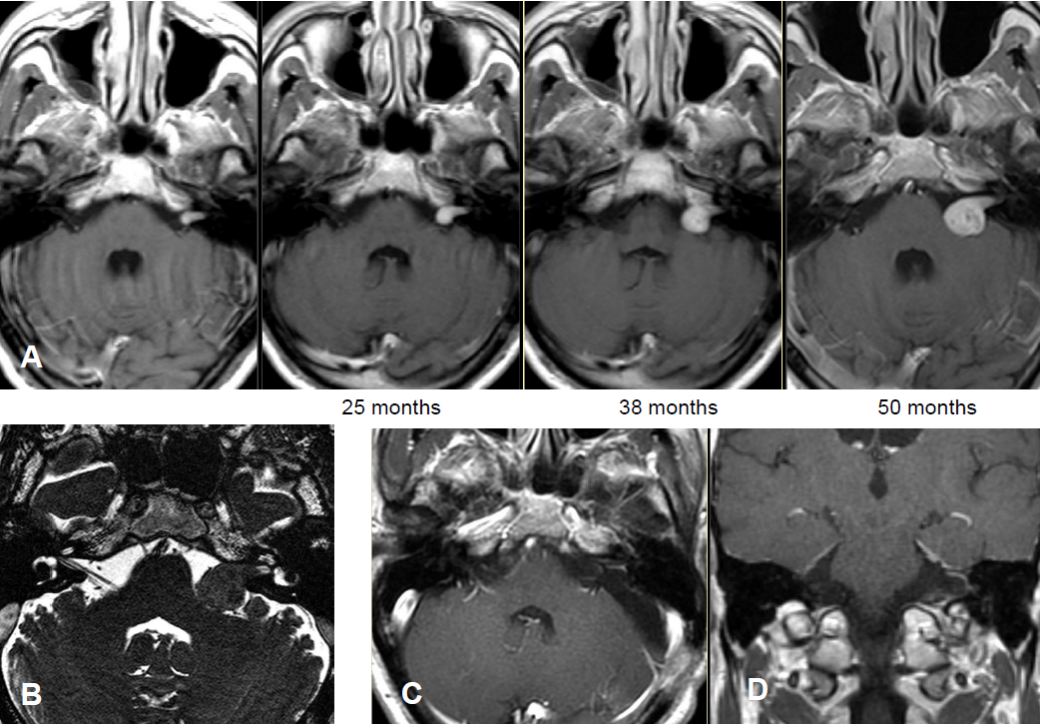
Upper row: MRI follow-up over more than 4 years; A T1-w + CM axial; lower roe: B T2 (FIESTA) transversal before surgery; T1-w + CM; C axial and D coronal postoperative.
